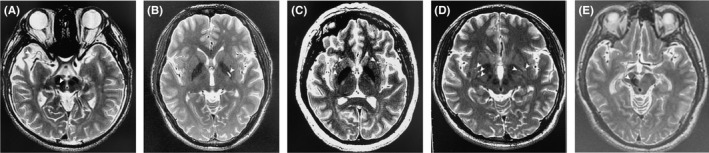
Preoperative brain MR images (T2‐weighted sequence) showing structure changes in the right midbrain and temporal lobe (A), the left globus pallidus (GP) (B), the bilateral external capsule (C), the bilateral globus pallidus (GP) and putamen (D), and the right midbrain (E). The location of the lesion is marked by a white arrow in each patient
